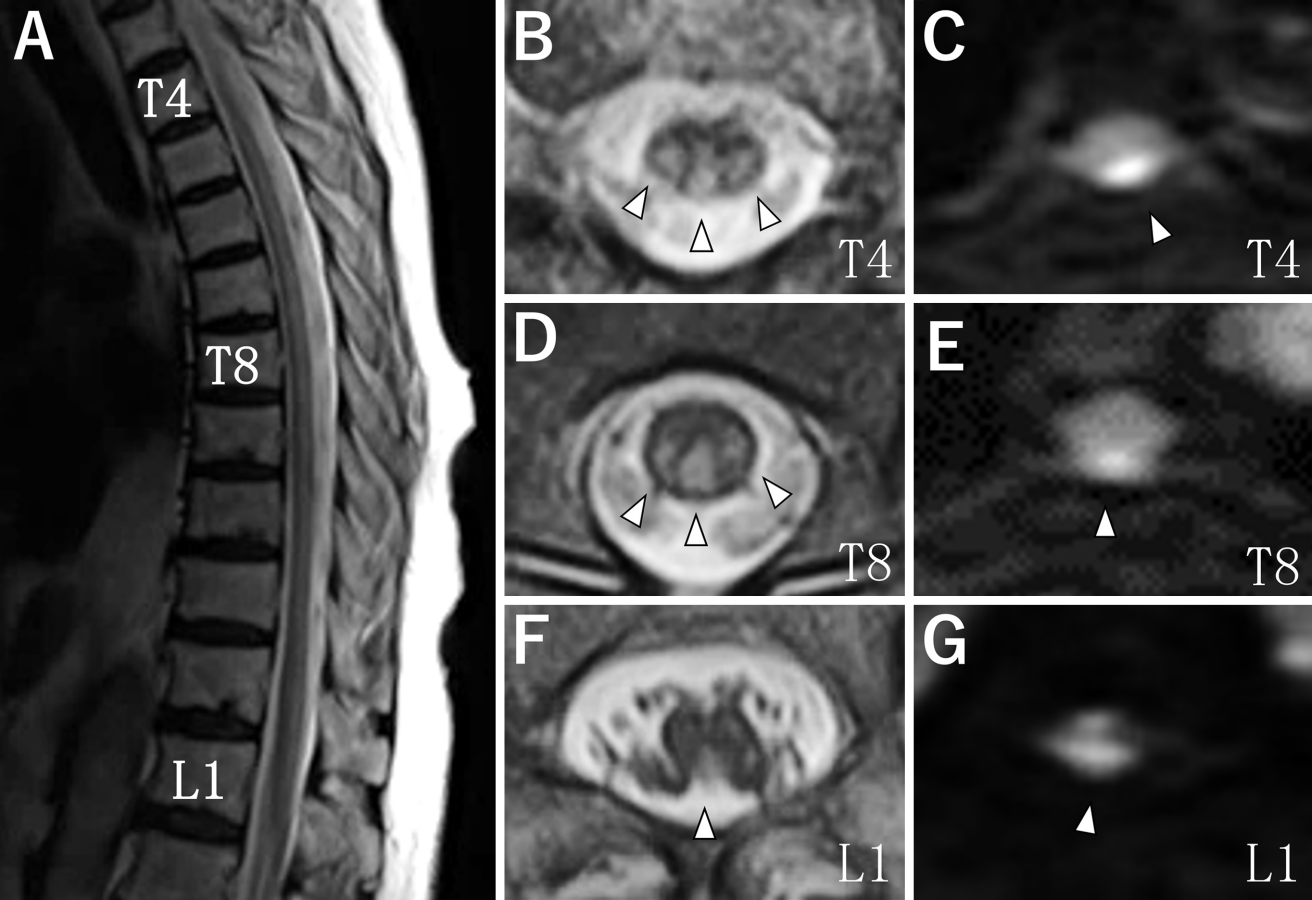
Spinal magnetic resonance imaging. Sagittal T2-weighted imaging (T2WI) showed longitudinal hyperintensity from vertebral level T4 to the conus medullaris of the spinal cord (A). Axial images showed hyperintensity in the posterior part of the spinal cord on T2WI and diffusion-weighted imaging (DWI) (B-G arrowheads). T2WI (B, D, F). DWI (C, E, G). The vertebral levels of each image are as follows: T4 (B, C), T8 (D, E), and L1 (F, G).
